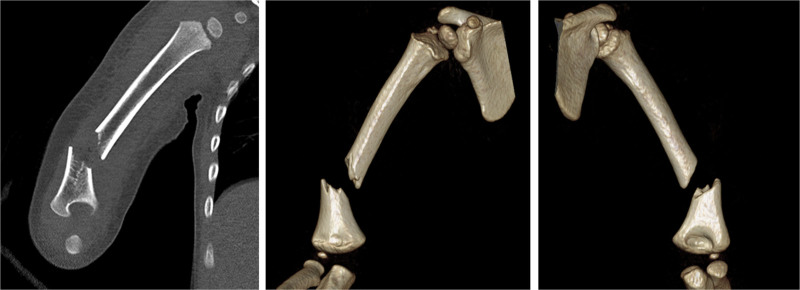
Computed tomography scans of the right humerus revealing a severely displaced metaphyseal–diaphyseal fracture of the distal humerus.
